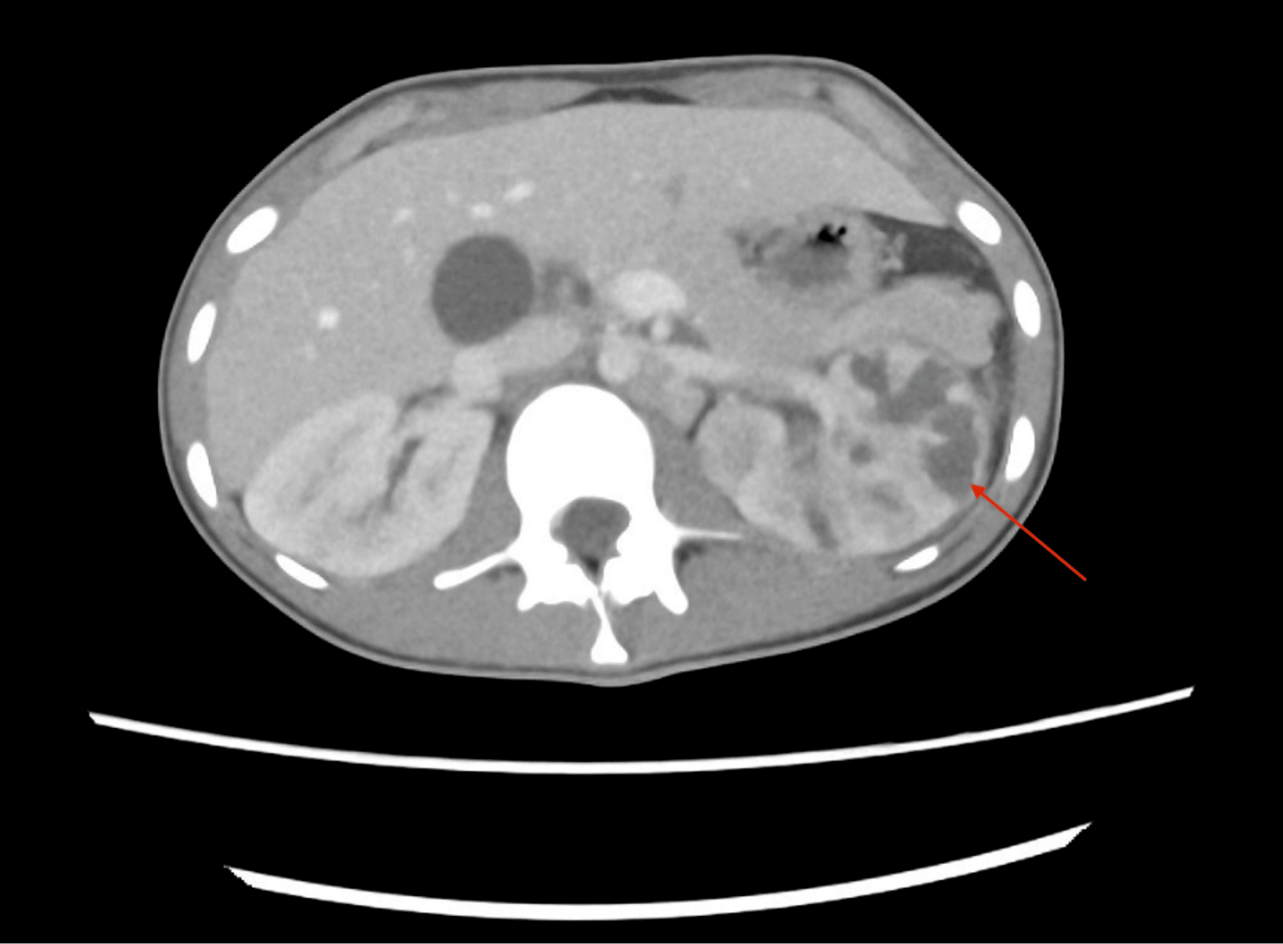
Contrast-enhanced scan of the abdomen in the axial view showing features suggestive of pyelonephritis CT: computed tomography
